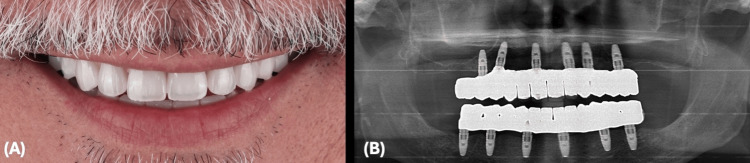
Clinical Outcome (A) Patient's smile at delivery. (B) Final panoramic radiograph.
